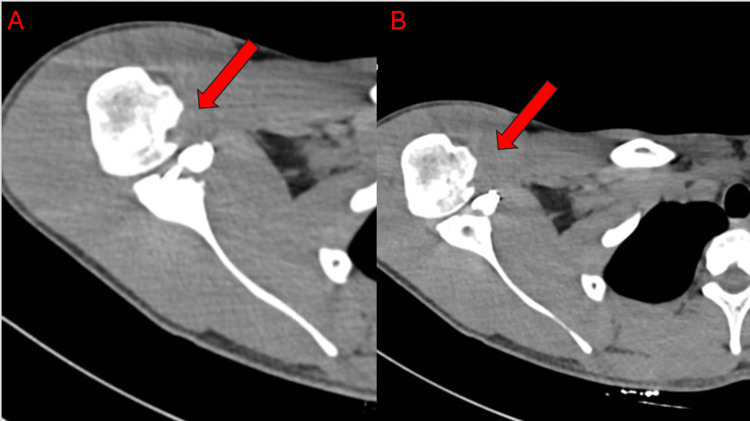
MRI of the right shoulder demonstrating significant anterior glenoid bone loss associated with recurrent anterior shoulder dislocations. Axial MRI images progressing from superior (A) to inferior (B) levels demonstrating a concomitant superior labral tear in the anterior-posterior plane lesion, Hill-Sachs deformity, and bony Bankart lesion associated with recurrent anterior shoulder instability.
